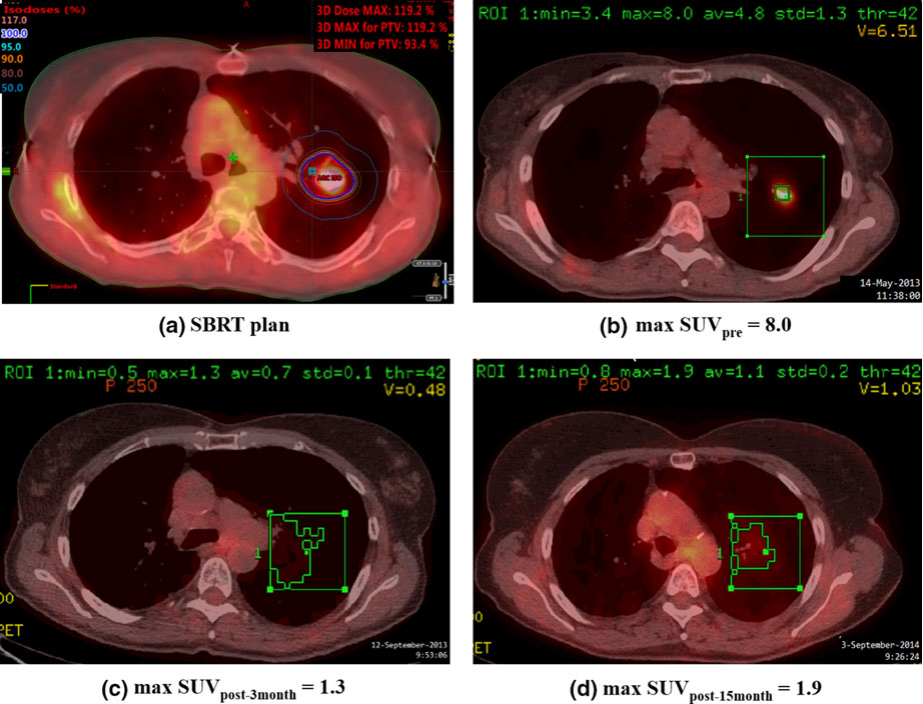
(a) 11 Gy × 5 SBRT plan; (b) PET/CT before SBRT, SUV
